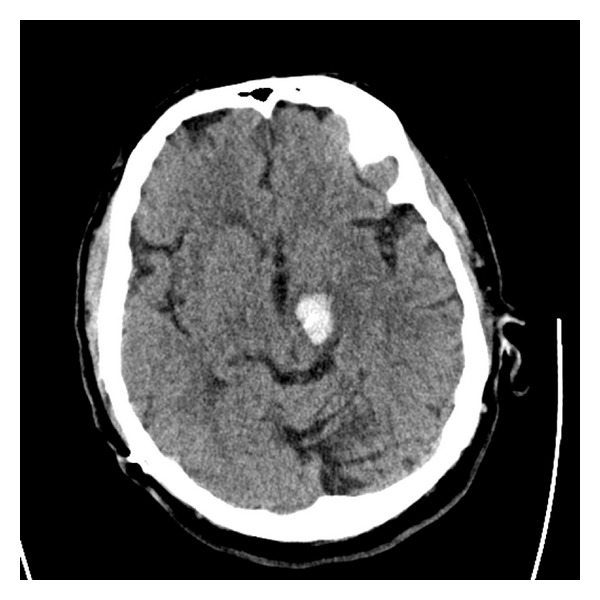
Axial CT without contrast enhancement showing 1.9 × 1.5 cm acute hemorrhage in the left cerebral peduncle five months after DBS placement.
